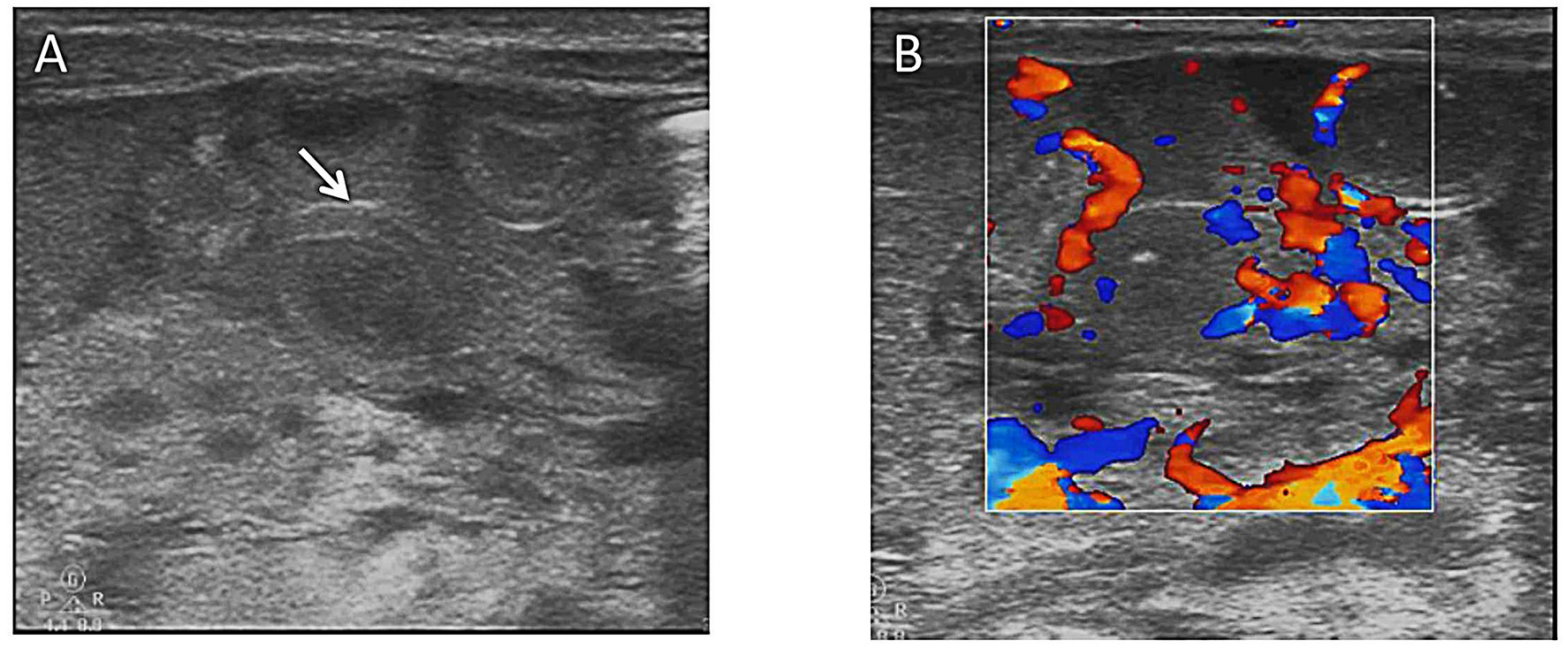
(A) Bowel wall thickening (arrows) on abdominal ultrasound. (B) Colored ultrasound revealed increased bowel wall perfusion.
